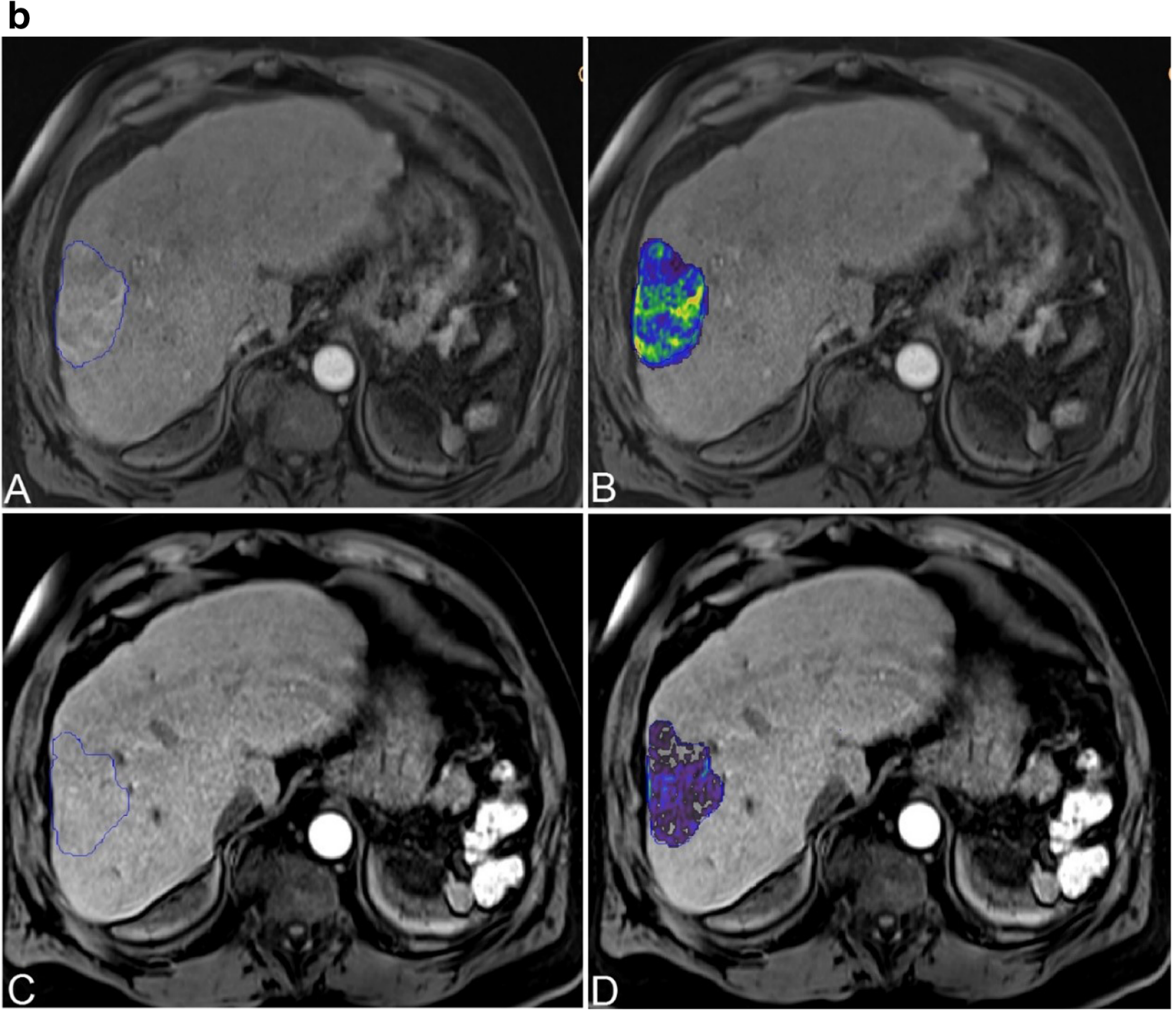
Quantification of total tumor volume and enhancing tumor volume on baseline and follow-up imaging. a Patient with highly vascularized HCC. Images only show representative tumor measurements in one layer. Target lesions were volumetrically assessed. A Preinterventional MRI: tumor tissue appears highly vascularized compared to surrounding liver parenchyma. B Colored enhancing tumor volume/total tumor volume (ETV/TTV) overlay. C Postinterventional MRI: the tumor appears to be de-vascularized after treatment. D ETV/TTV overlay. b Patient with intermediately vascularized HCC. A Preinterventional MRI: Intermediate vascularization of tumor; relative arterial vascularization is color-coded in (B). C Postinterventional MRI: a tumor with initially intermediate vascularization loses hyper-vascularization compared to surrounding liver tissue. D ETV/TTV overlay
